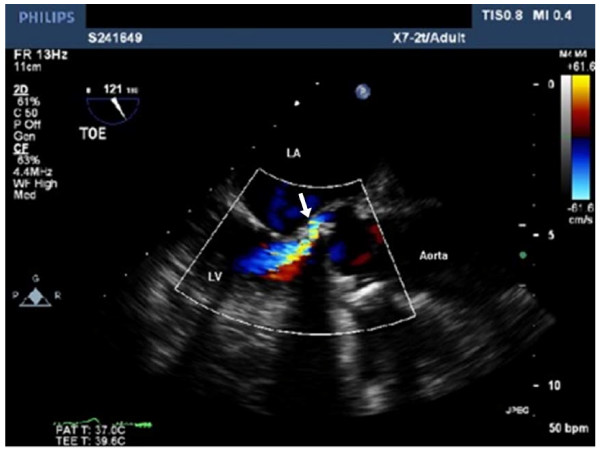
Pre-operative transoesophageal echocardiogram in the Left Ventricular Outflow Tract view. This appears to demonstrate an eccentric jet of aortic regurgitation (white arrow) from the posterior paraprosthetic margin of the aortic valve replacement.
